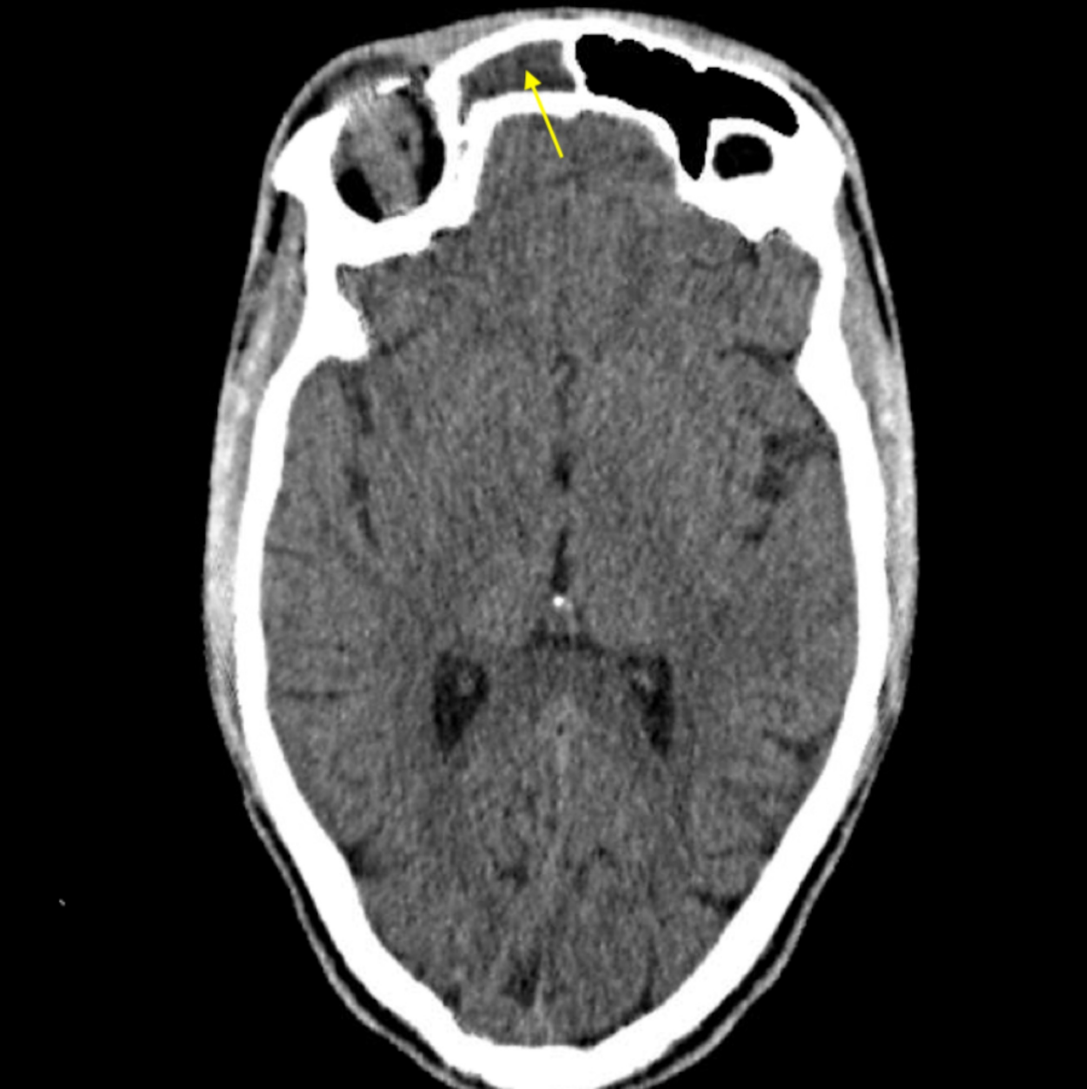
Opacification of the right frontal sinus on computed tomography of the head.
